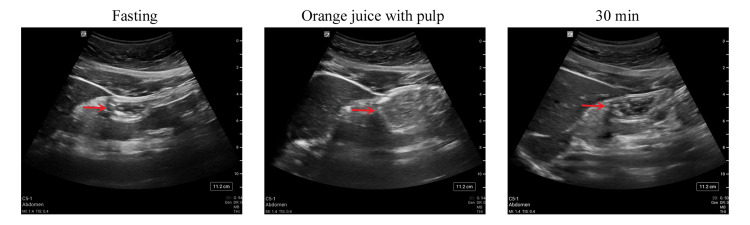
Ultrasonographic images of the gastric antrium Ultrasonographic images of the gastric atrium, as indicated by the red arrow, at fasting state, immediately after drinking orange juice with pulp, and 30 min afterward.
